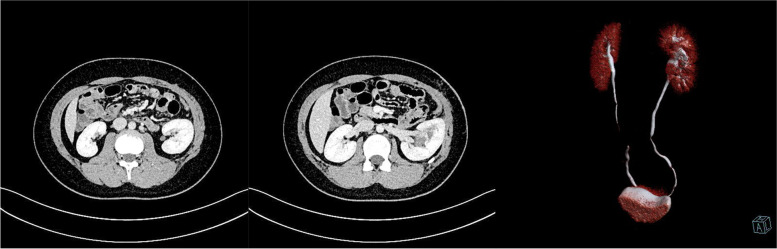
Seven-month postoperative urological CT. The left kidney was slightly larger, with irregular morphology of the superior parenchyma, slightly enlarged local renal pelvis, and normal renal structure, considering postoperative changes
